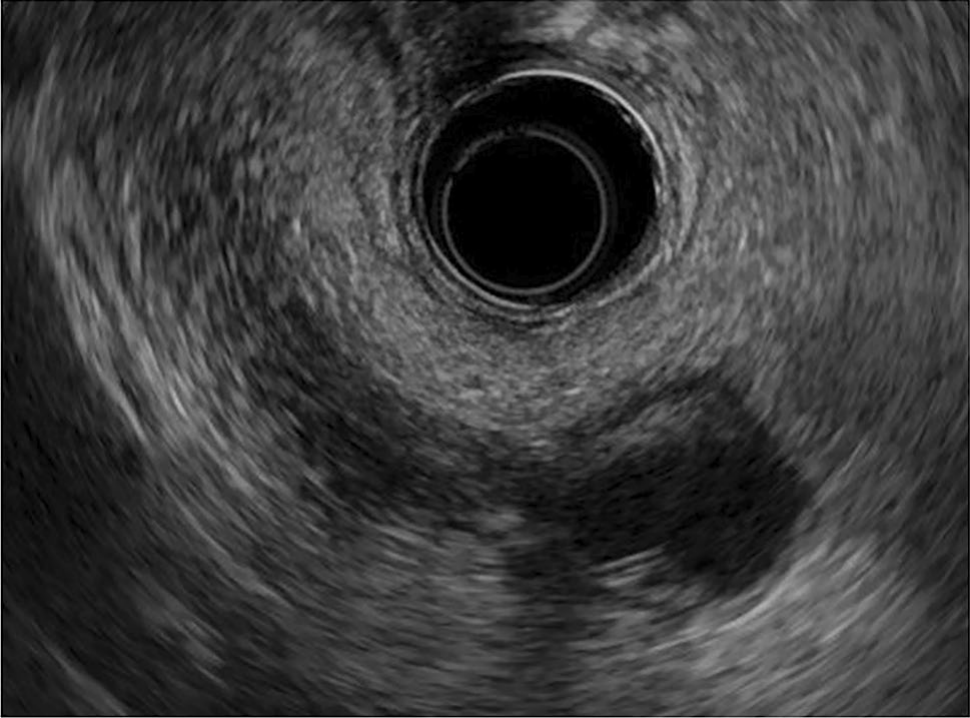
Endoscopic ultrasonography. A gourd-shaped, well-demarcated, internally anechoic cystic lesion with a three-layered wall was observed in the pancreatic tail
